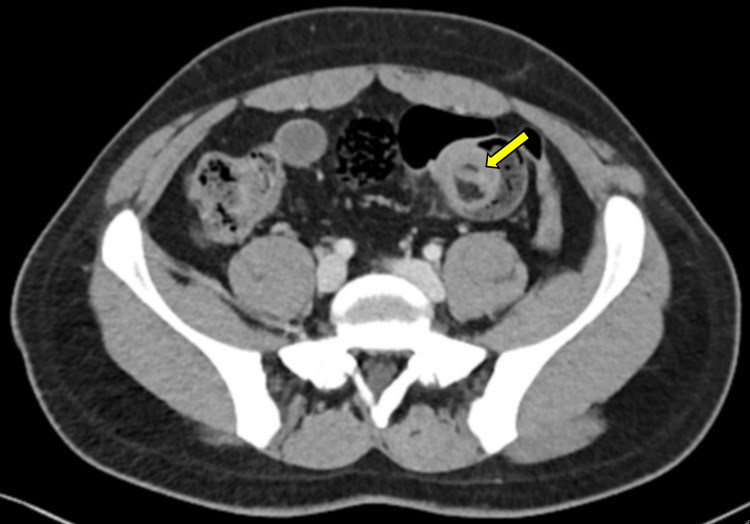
CT imaging of ileo-ileal intussusception. Axial contrast-enhanced CT scan (portal venous phase) demonstrating ileo-ileal intussusception with a central fat-density lesion (arrow, approximately -80 to -120 Hounsfield units), consistent with a submucosal lipoma acting as the lead point. Note the "target sign" with invaginated mesenteric fat and vessels, without evidence of bowel ischemia or perforation.
